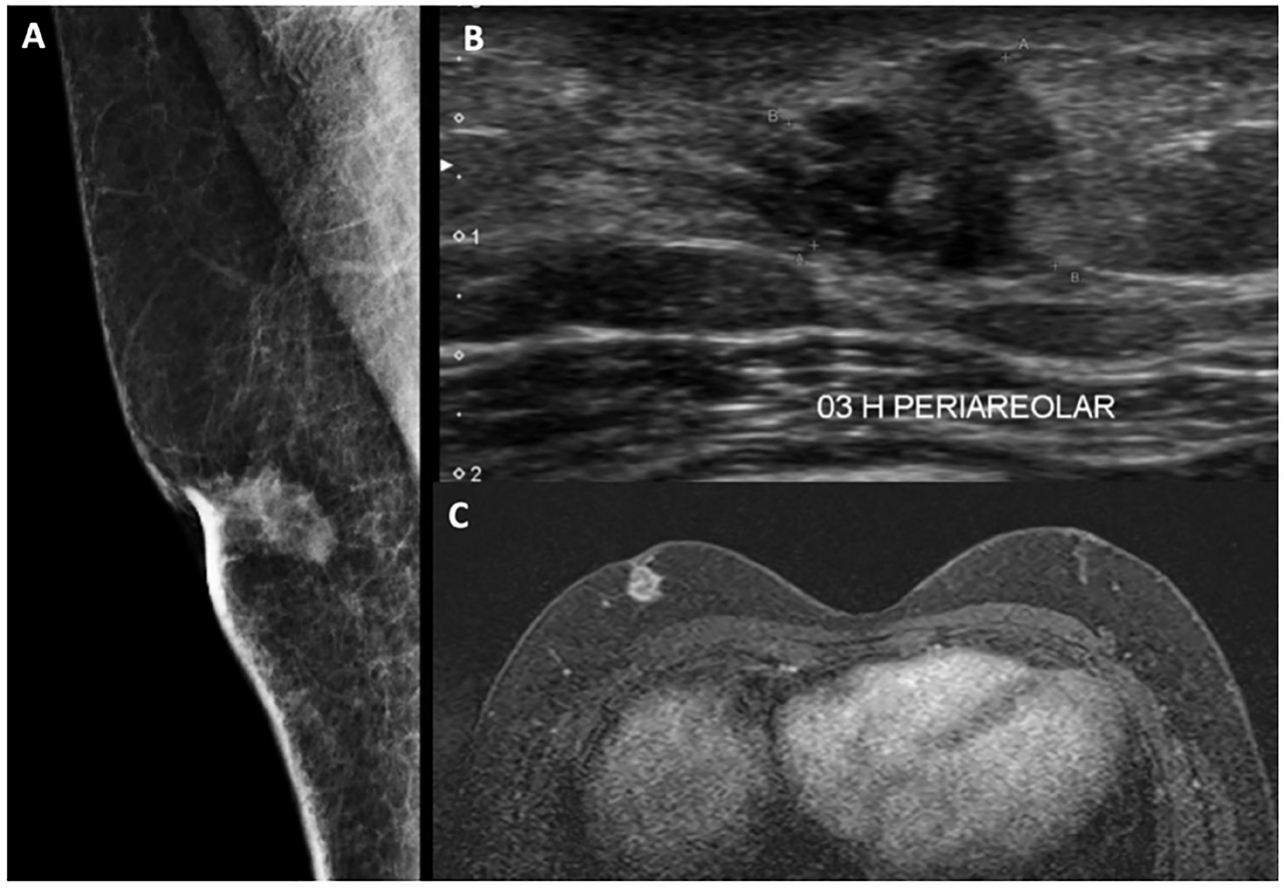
An irregular mass in the retroareolar region of the right breast in a 44-year-old man, seen on mammography (A), ultrasonography (B), and magnetic resonance imaging (C).
